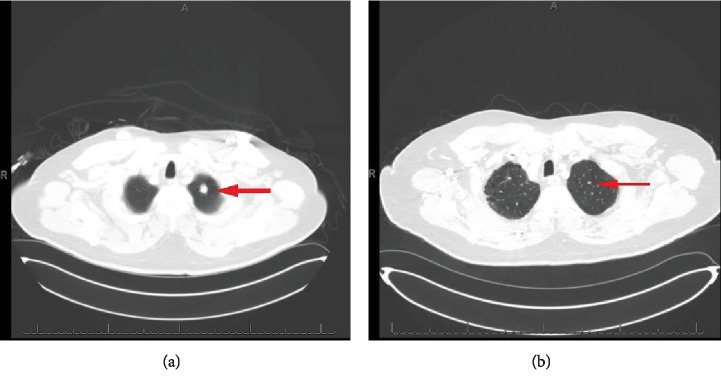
(a) CT chest w/ contrast. Arrow showing a 1.4 cm peripherally enhancing, partially speculated nodule in the left upper lung apex. (b) CT chest w/ contrast; ~18 months post discontinuation of nivolumab with the following findings: arrow showing a 0.4 cm enhancing nodule in the left upper lung lobe.
